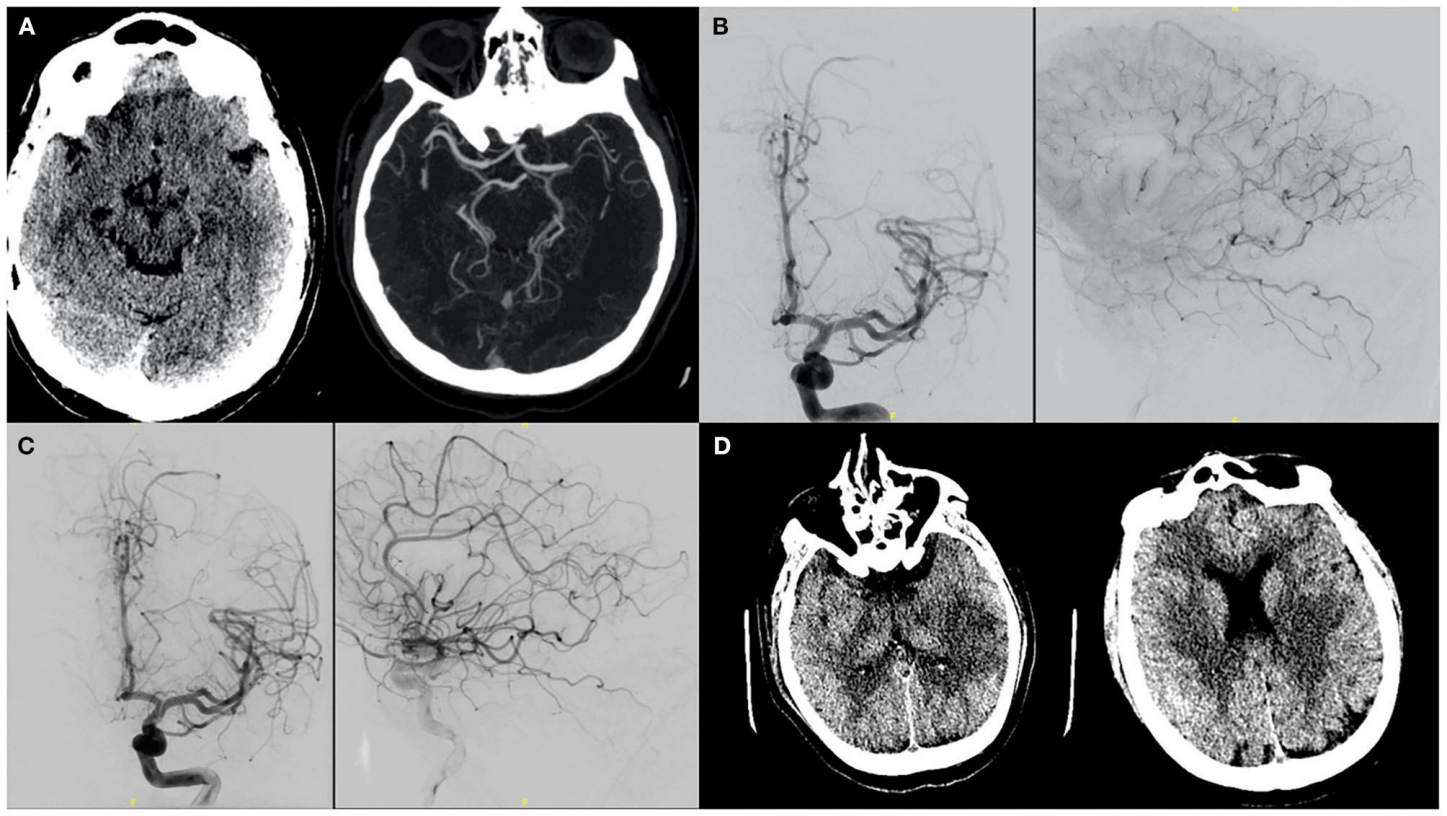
A patient with no significant past medical history presented to the ER after 8 days of viral illness and was on Azithromycin therapy after testing positive on day 3. NIHSS was 18 on presentation secondary to a left MCA syndrome. (A) CT/CTA confirmed a left M1 thrombus. As presentation was within 4.5 h of symptoms onset, IV tPA was administered. (B) The patient was taken for mechanical thrombectomy and on initial cerebral angiography showed partial recanalization post-TPA and migration of the clot to distal MCA branches. (C) The patient was thus given intra-arterial tPA in these branches. (D) Post procedurally, infarcts in the left putamen as well as temporal and parietal regions were seen. On last follow-up patient's mRS was 3.
